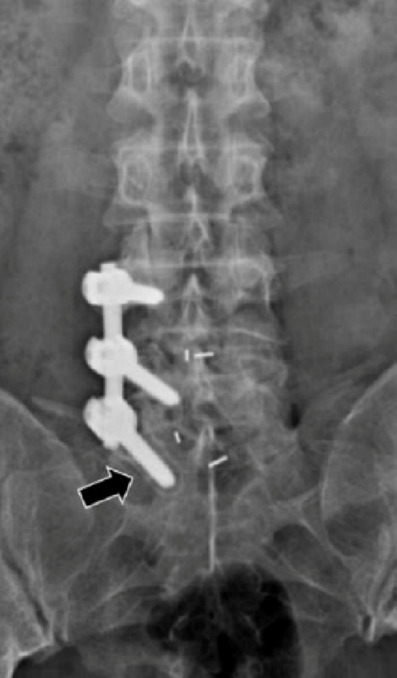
The screw loosening was occurred in surgery of unilateral pedicle screw fixation (UPSF) combined with interbody fusion (IF). Male, 59 years old, preoperative diagnosis was L4-S1 lumbar spinal stenosis (LSS). Anteroposterior radiographs of the follow-up 4 years after the surgery indicates screw loosening at caudal side (radiolucent line around the implant > 1 mm wide).
